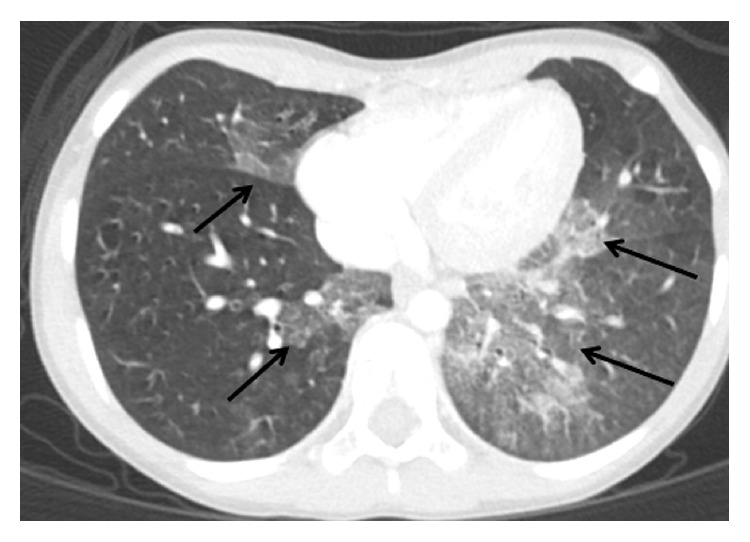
High resolution CT image of lower lobes. Arrows show diffuse hazy mixed alveolar interstitial infiltrates involving much of the left lower lobe and medial portions of the lingula, right middle lobe, and medial right lower lobe.
